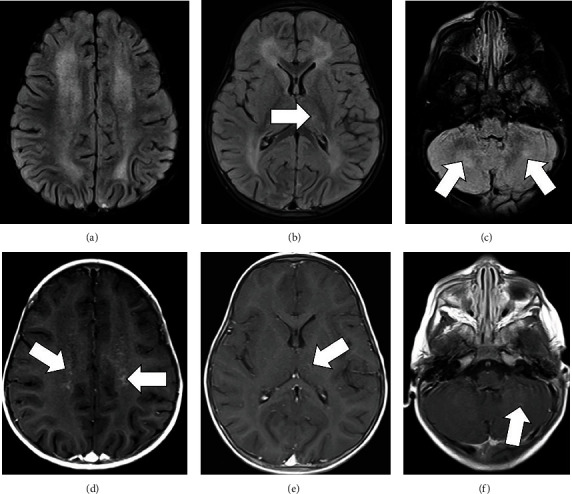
MRI obtained on admission demonstrating diffuse confluent symmetric T2 FLAIR hyperintensities in the supratentorial periventricular and deep white matter, sparing the subcortical U fibers, and in bilateral cerebellar hemispheres around the deep nuclei (a–c). A focus of T2 FLAIR hyperintensity is noted in the lateral left thalamus (arrow in (b)). Postcontrast images reveals punctiform and curvilinear enhancement centered in bilateral supratentorial periventricular white matter with a perivascular distribution pattern (arrows in (d)). Foci of faint and curvilinear enhancement (arrow in (f)) were also present in bilateral cerebellar white matter. Small curvilinear enhancement was noted in the lateral left thalamus, likely reflective of a vein or a perivenous process (arrow in (e)).
